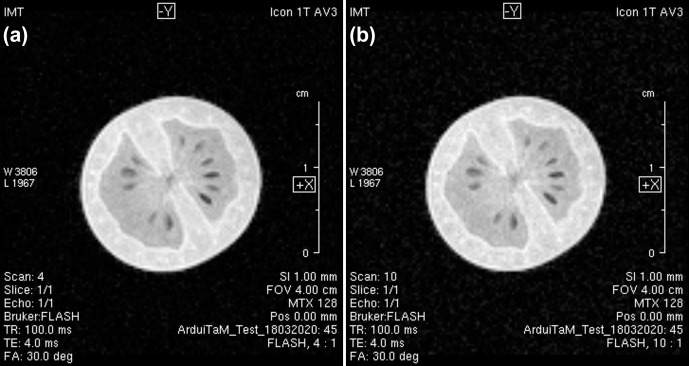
Integrating the ArduiTaM with a commercial MRI scanner shows only a negligible effect on its performance. (a) MR image of a cherry tomato before applying the ArduiTaM. The measured SNR is 49. (b) Repeating the same experiment after applying the ArduiTaM. The measured SNR here is 43, reflecting a loss of approximately 12 %, due to the insertion loss of the RF switch (ZFSWA-2-46 from Mini-Circuits^®^).
